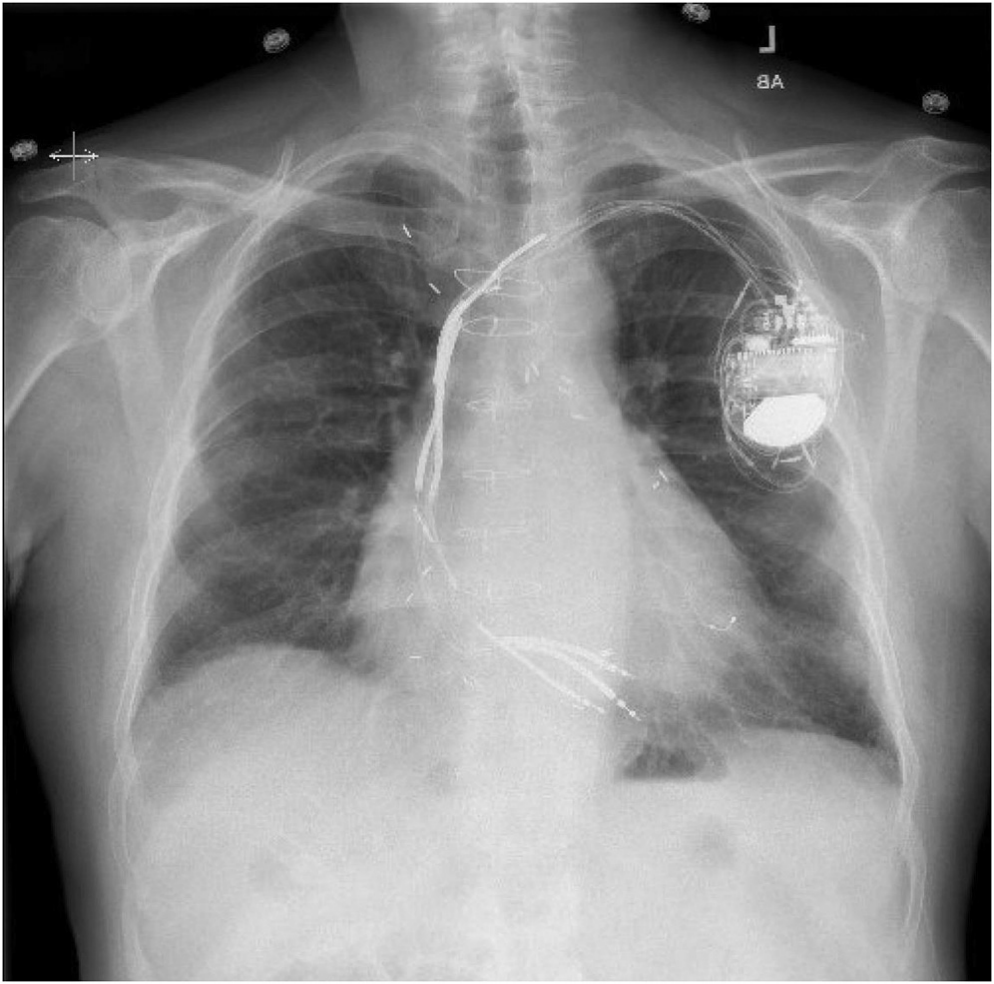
Preprocedural chest radiograph of biventricular implantable cardioverter-defibrillator with active and abandoned leads.
